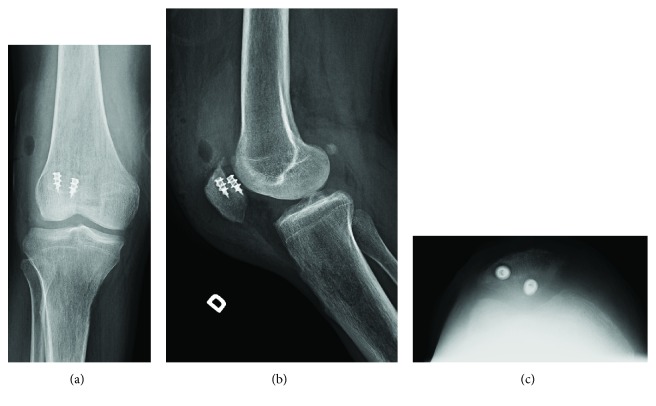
Postoperative (a) AP, (b) lateral, and (c) sunshine view of X-rays of the knee joint, showing 2 anchors in the proximal patella.
